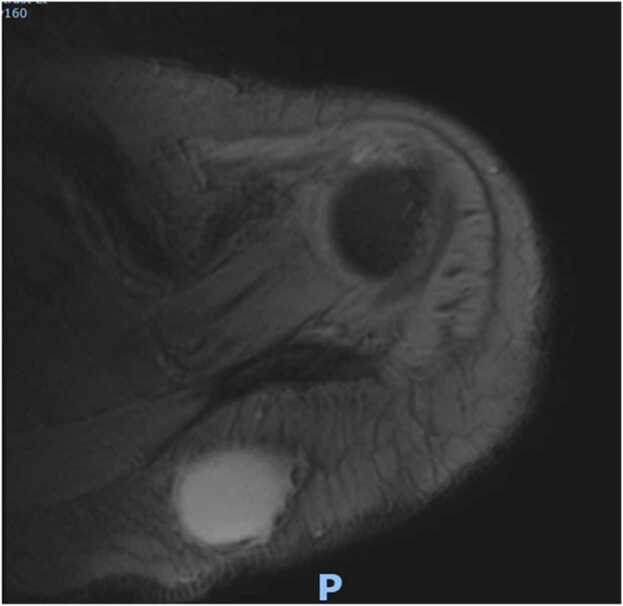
Precontrast and post contrast MRI images of patient performed at 2 weeks.
